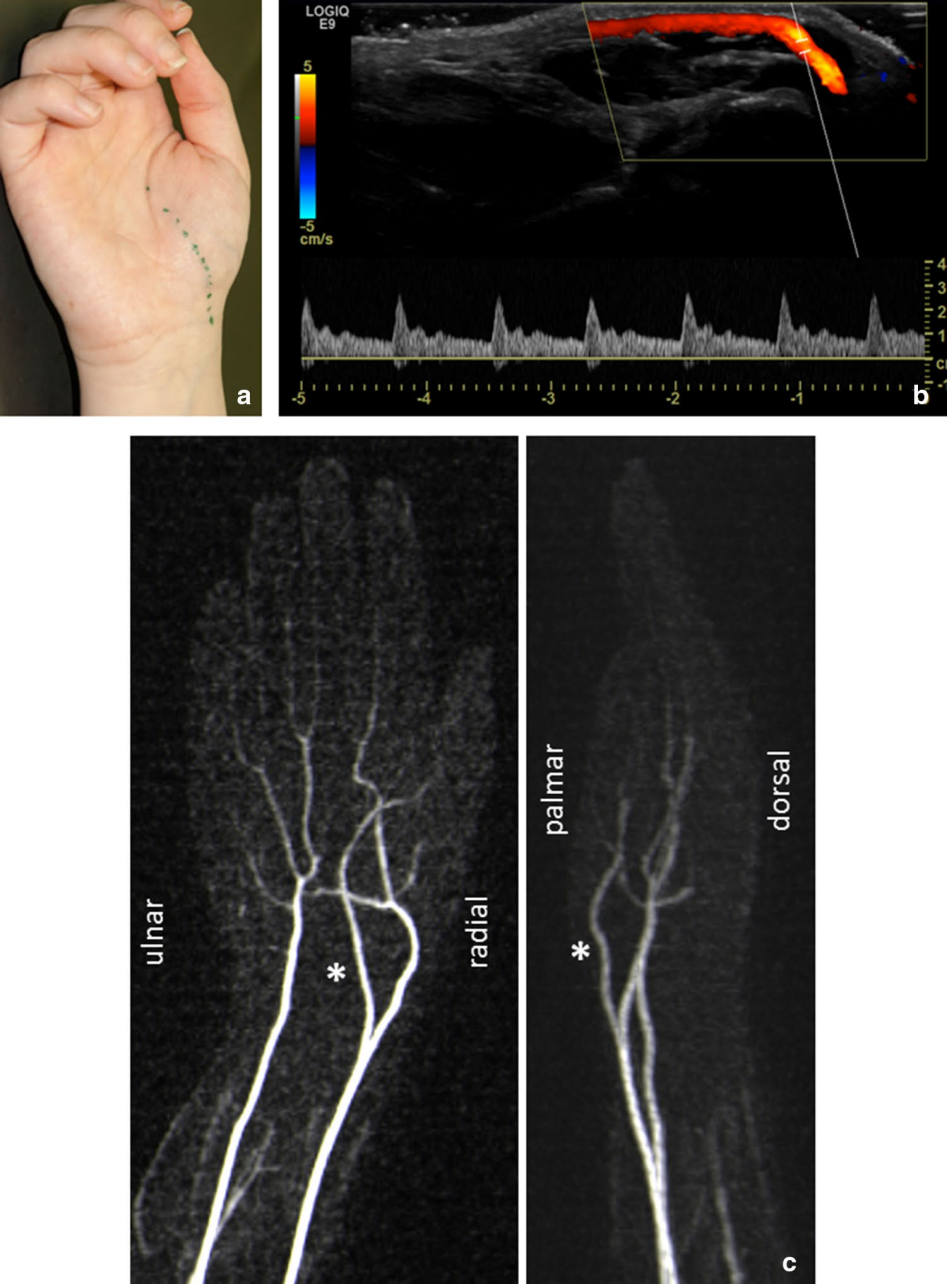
Palpable course of the superficial branch of the radial artery (a). Duplex sonography revealed a subcutaneously located vessel with arterial flow pattern (b). Magnetic resonance angiography demonstrated a large calibered SPBRA with a superficial course (asterisk, c)
